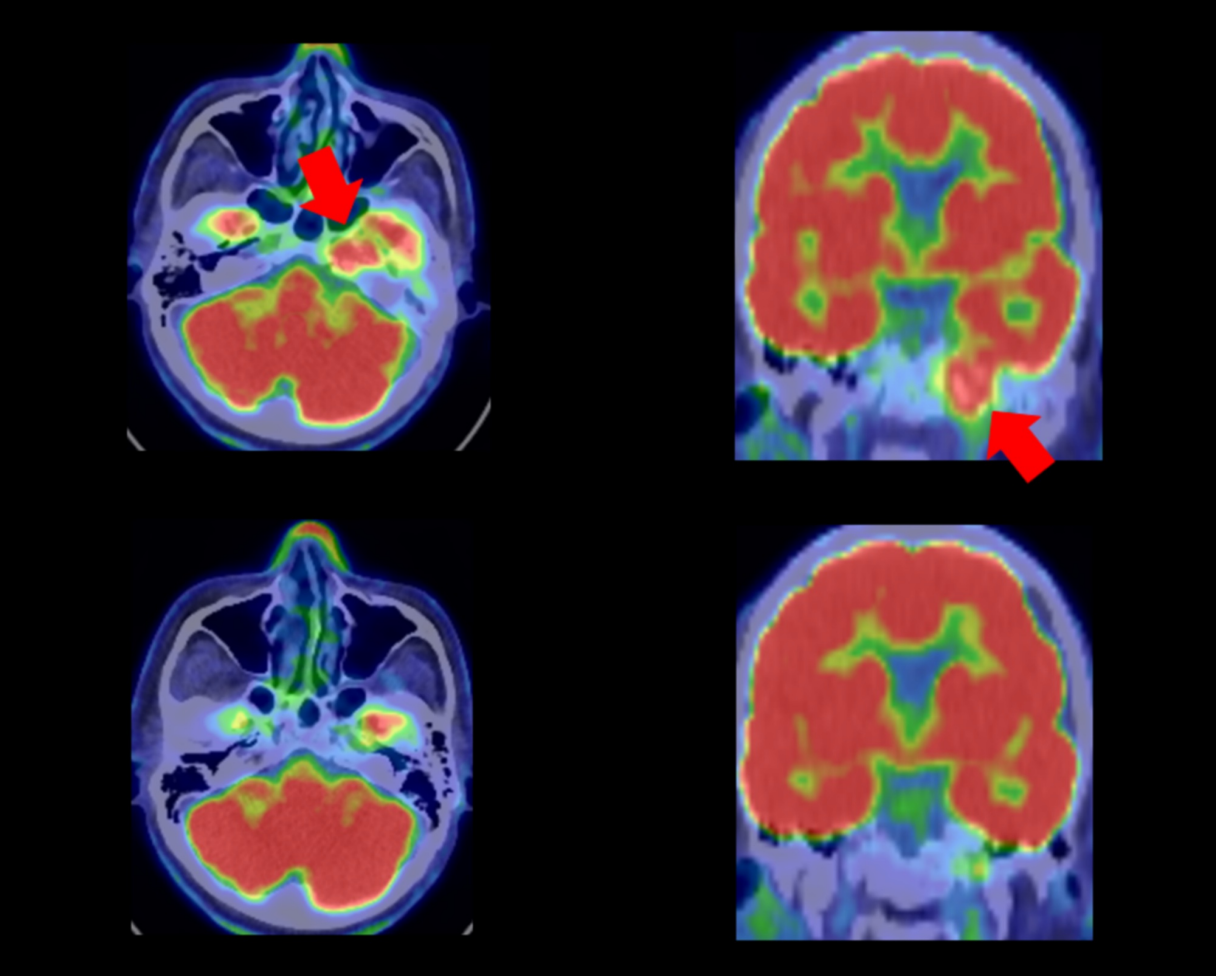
FDG-PET of Case 1 FDG-PET before CyberKnife (top) and after CyberKnife (bottom). The tumor (red arrow) disappeared after treatment. FDG-PET: fluorodeoxyglucose-positron emission tomography
